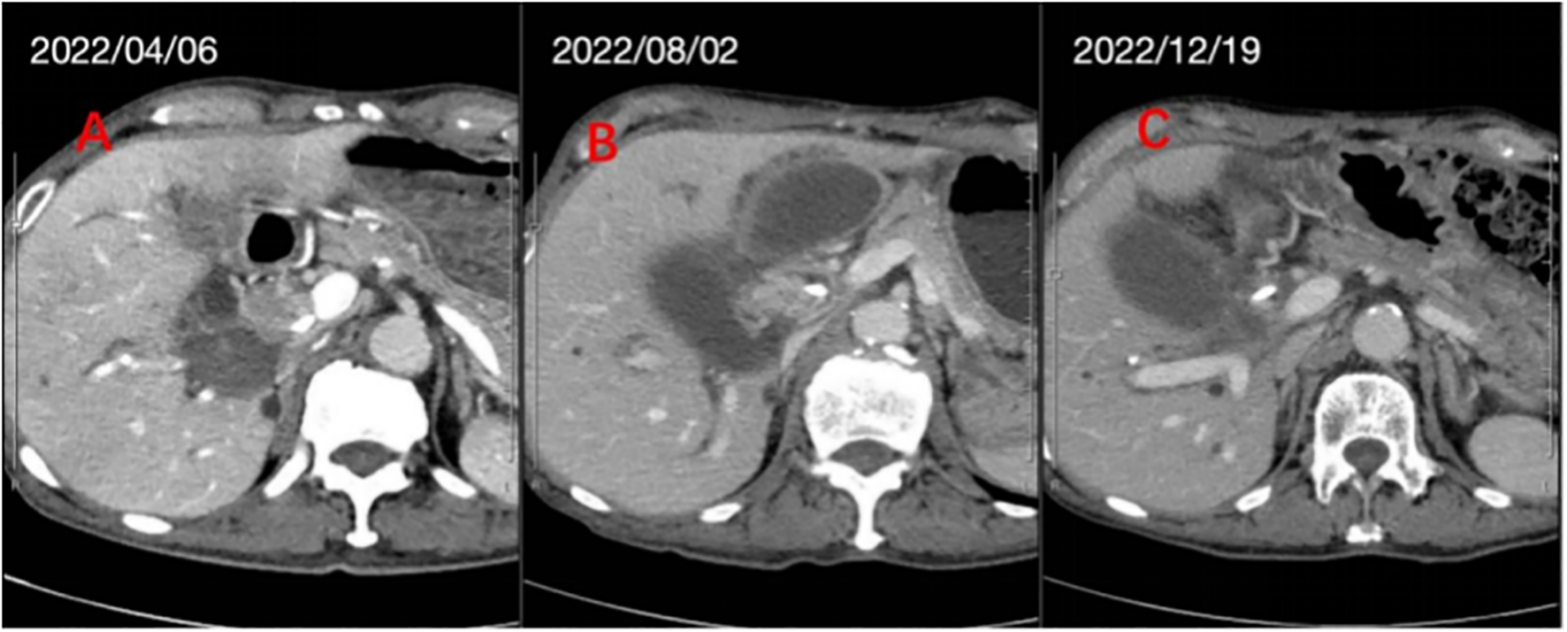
CT images before treatment (A), and at 1 month (B) and 5 months (C) after the proton therapy.
